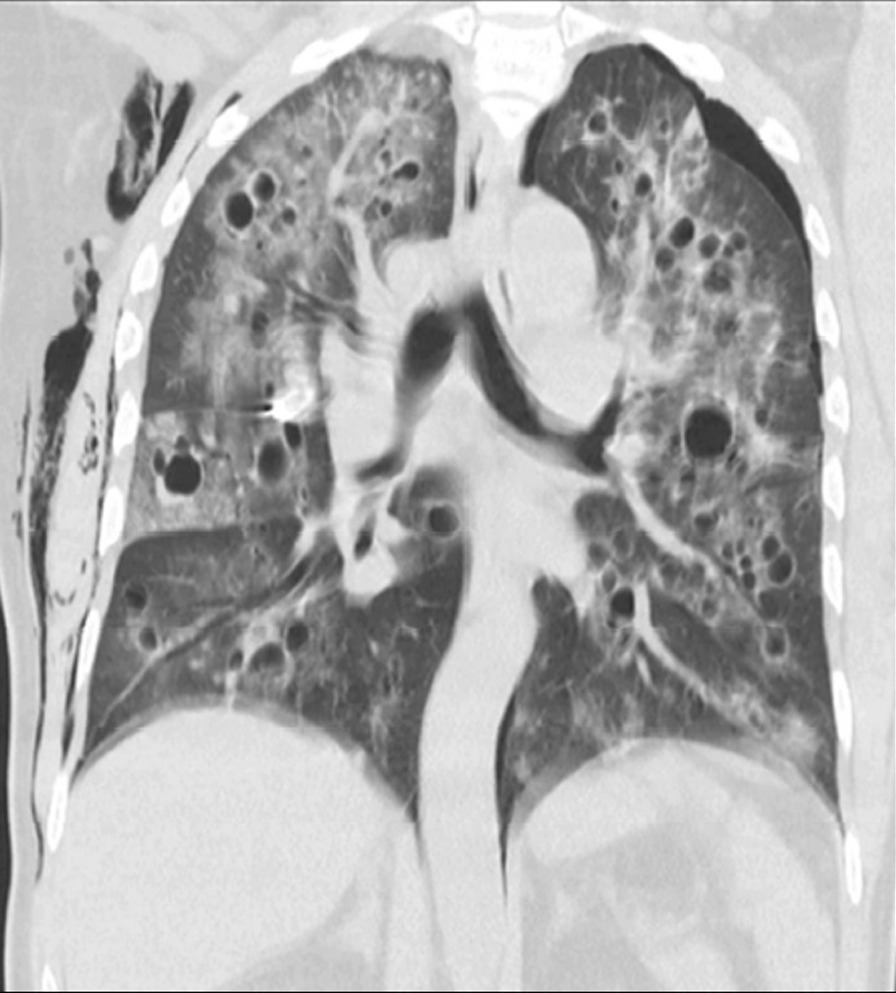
High resolution computed tomography (HRCT) Chest showing multiple cystic lesions bilaterally, patchy areas of ground glass opacities and nodules with bilateral pneumothorax
